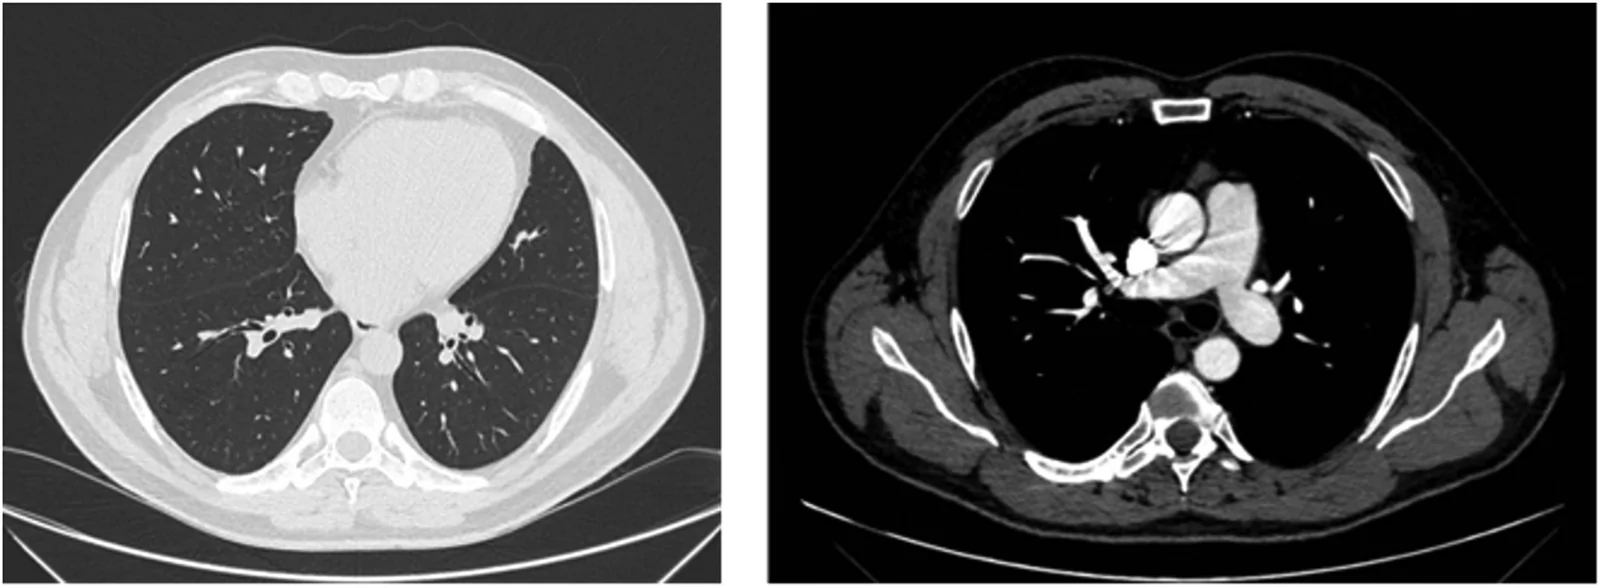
Chest computed tomography documenting normal lung parenchyma and absence of thromboembolic disease of the pulmonary vasculature.
